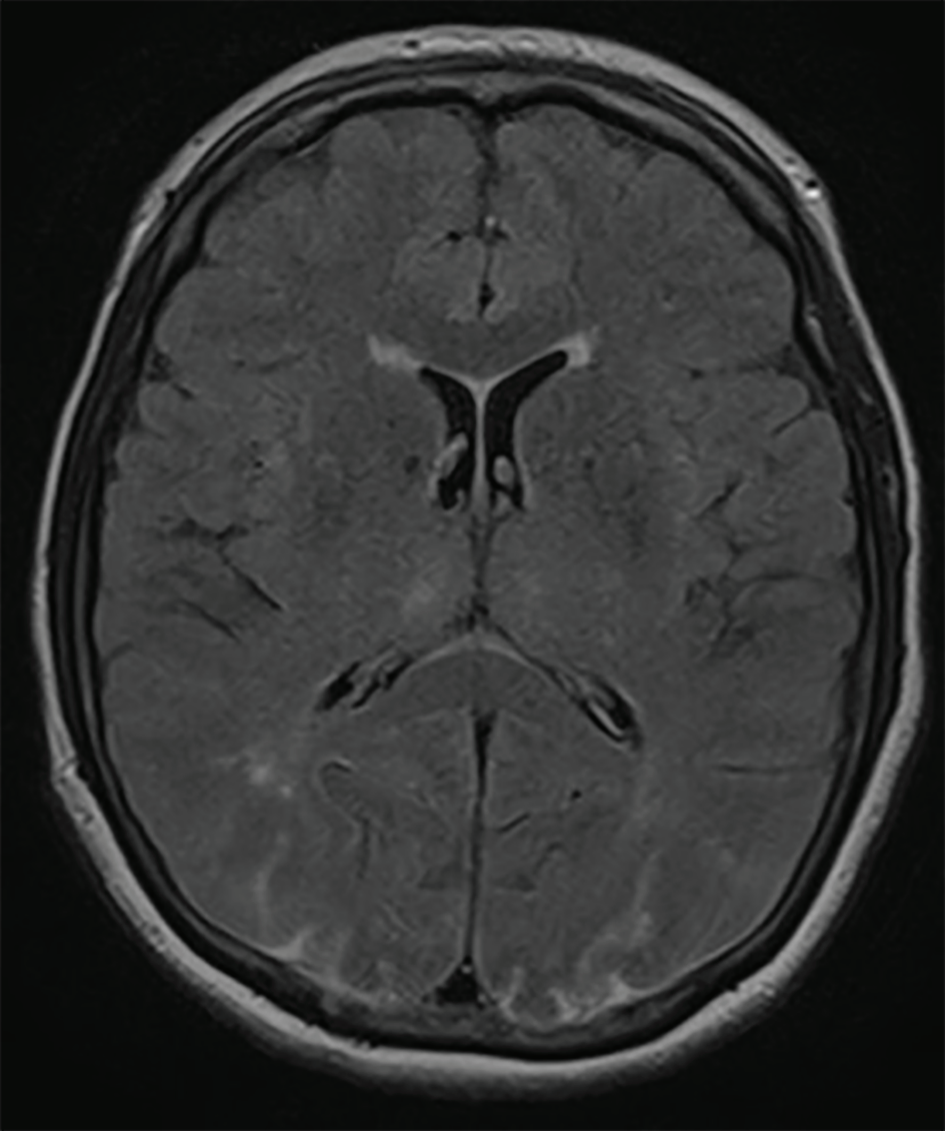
Axial FLAIR MRI demonstrating improvement in the hyperintense lesions in both occipital lobes 48 h after symptom onset.
